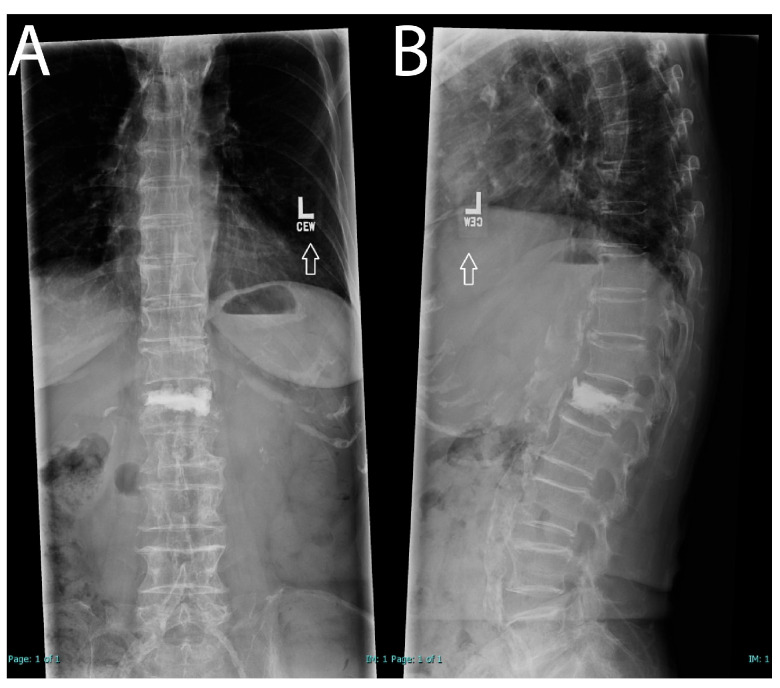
One-month postoperative X-ray (A,B); X-ray AP (A); X-ray lateral (B). Arrowheads are markers from the original imaging acquisition and are not present for demonstration purposes.
